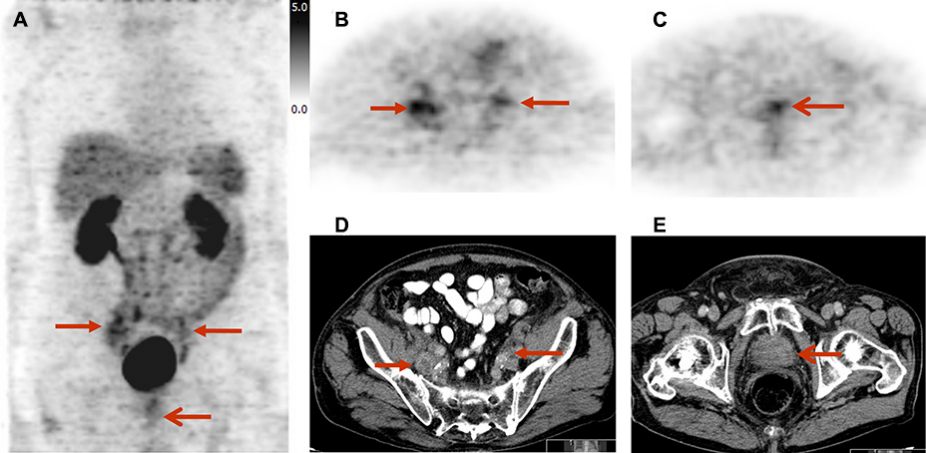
Imaging example of lymph node metastases and primary tumor site in a 82 year old patient with prostate cancer under androgen deprivation therapy (initial staging cT3a, cN1, cM1; Gleason score 7; PSA 146.2 ng/ml): lymph node metastases (B, D) show a slightly to moderately elevated, heterogeneous uptake of [^18^F]Galacto-RGD; the primary tumour site (C, E) shows a slightly elevated uptake Due to a generally higher level of background activity in the intestine and urinary system, detection of tracer uptake in lymph node metastases was more difficult. MIP [^18^F]Galacto-RGD PET (A), PET (B) and CT (D) showing lymph node metastases, PET (C) and CT (E) showing primary local tumor site. Arrows indicating lymph node metastases and primary local tumor site.
